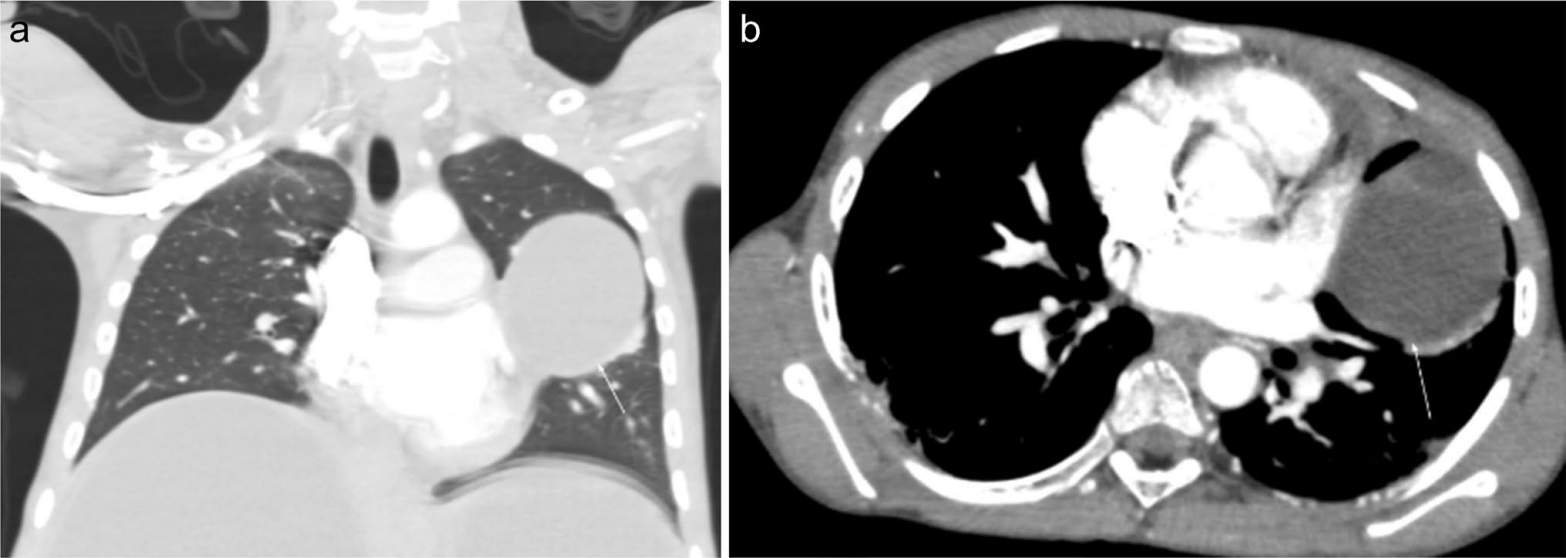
Contrast-enhanced computed tomography images in an 11-year-old boy with hydatid disease who presented to hospital with a 2-month history of hemoptysis, abdominal distention, and elevated inflammatory markers. The patient had a history of travel to an endemic area in the 5 months prior to presentation. Coronal lung window (a) and axial mediastinal window (b) images demonstrate a well-defined cystic mass (*arrows*) in the mid zone of the left lung. The cyst wall has no calcification and enhances after contrast agent injection, while the content of the cyst remains homogenously hypodense
